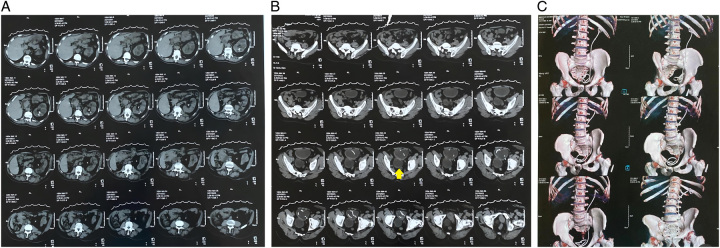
Non-contrast computed tomography (NCCT) abdomen and pelvis showing a non-visualized right kidney and an enlarged left kidney with a percutaneous nephrostomy *in situ*, moderate calyceal dilatation, air foci within the calyces, stranded perinephric fat, and no stones. DJ stent was seen extending from the left pelvicalyceal system (A) into the left ureter, which otherwise had a normal course with no stones, and later into the urinary bladder (B). The urinary bladder was also seen showing a 7 cm diverticulum (arrow), stranded peri-vesical fat reflecting infection (cystitis), and no stones. The three-dimensional reconstructed NCCT showed what would be a course of the existing left ureteral stent extending from the left pelvicalyceal system into the left ureter and eventually the urinary bladder (C).
